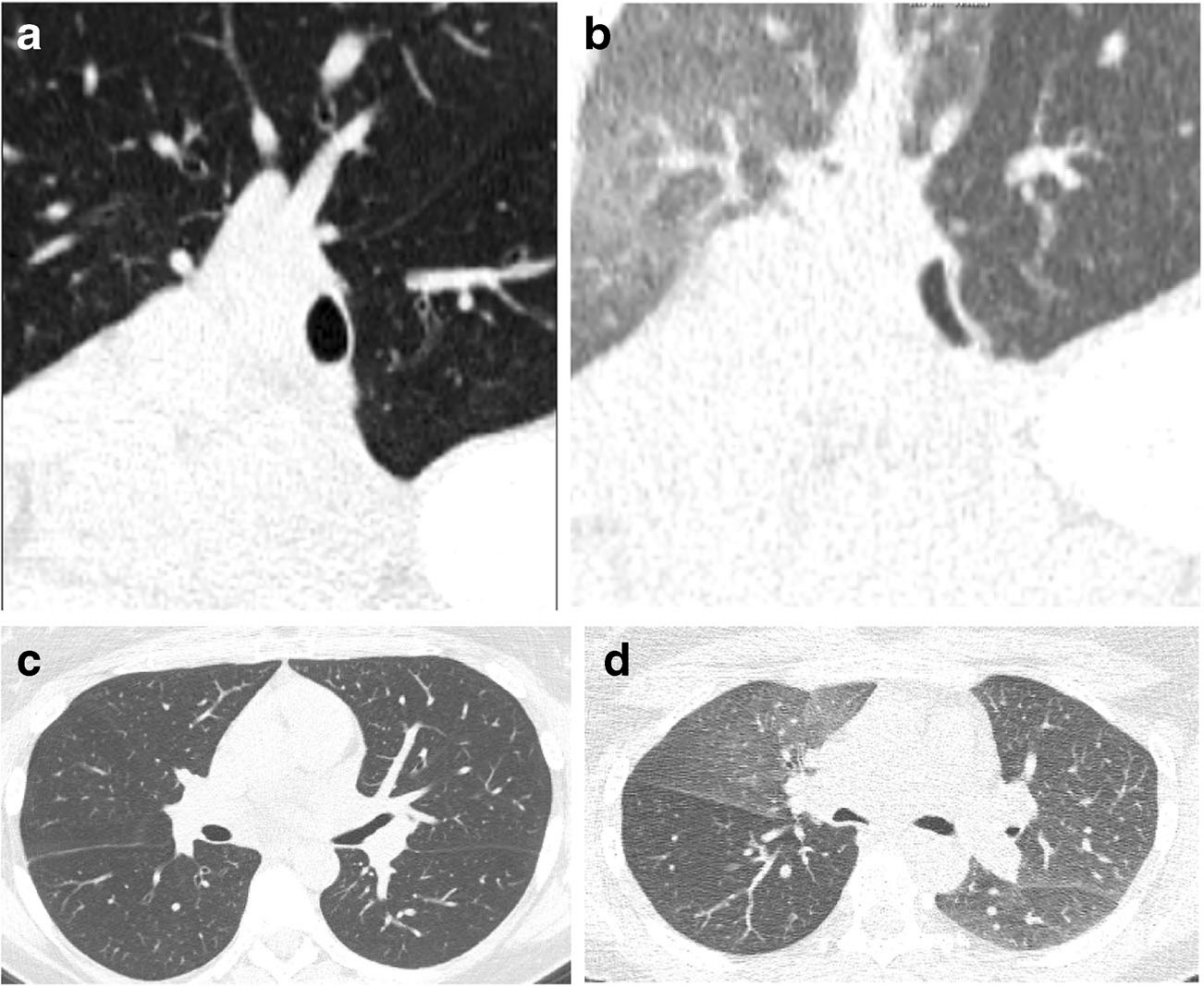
Thirty-four-year-old woman with history of atypical interstitial pneumonia status post bronchoscopy presenting with tracheobronchomalacia of the right bronchus intermedius. a Double oblique inspiratory and b expiratory CT imaging demonstrating reduced bronchial diameter on expiration. c Axial inspiratory CT imaging demonstrating air trapping on expiration d
